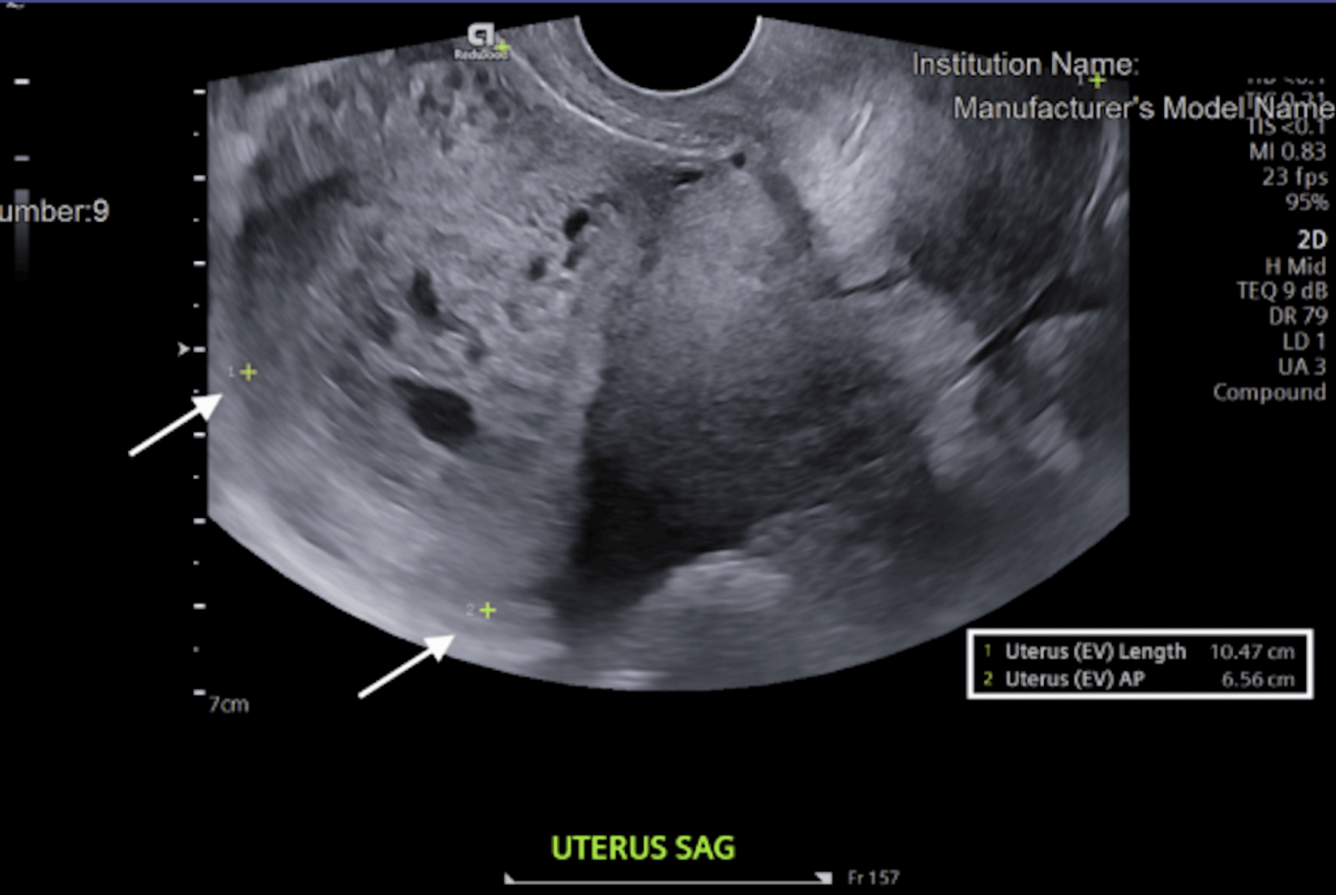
TVUS showing distortion and thickening of the endometrium with grossly abnormal heterogeneous and cystic components with suggested invasion into the myometrium TVUS: Transvaginal ultrasound
